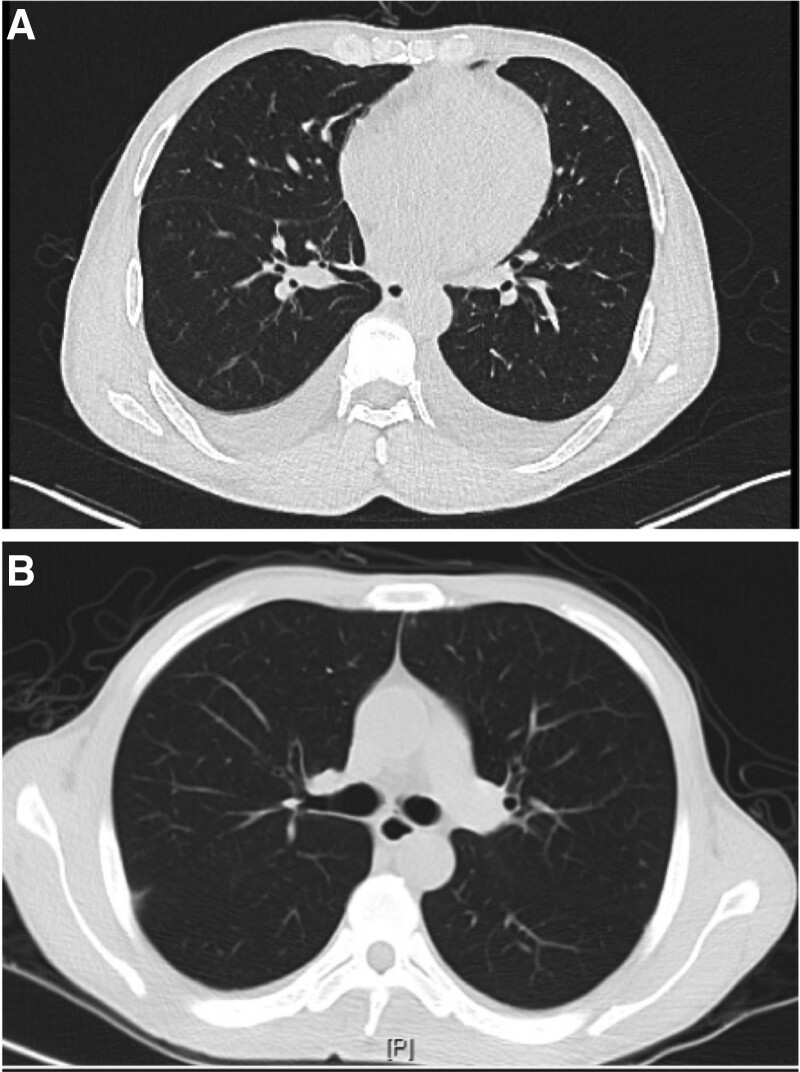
(A) Computerized tomography (CT) scan of further examination on admission, showing pleural effusion (arrowhead). (B) CT showed that the pleural effusion disappeared after treatment. CT = Computed tomography.
